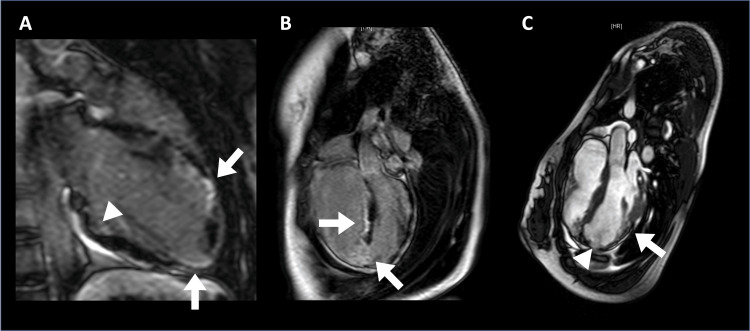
Cardiac magnetic resonance imaging of case 2 at diagnosis Left ventricular ejection fraction, 38%; cardiac index, 1.2 L/min/m² A) Late gadolinium enhancement image showing fibrosis and fatty replacement of the myocardium of the right ventricle (RV) with intense signal at the anterior wall and apex (bold arrows). The area pointed to by a triangle shows thinning of the myocardium of the RV; B) Late gadolinium enhancement image showing fibrosis and fatty infiltration of the myocardium also in the area including the apex and the interventricular septum (bold arrow); C) Sequence of gradient echo cine showing thinning of the myocardium of the area including the posterior wall (bold arrow) and apex (triangle) of the left ventricle. Aneurysm formation is also seen at the apex of the left ventricle (triangle).
